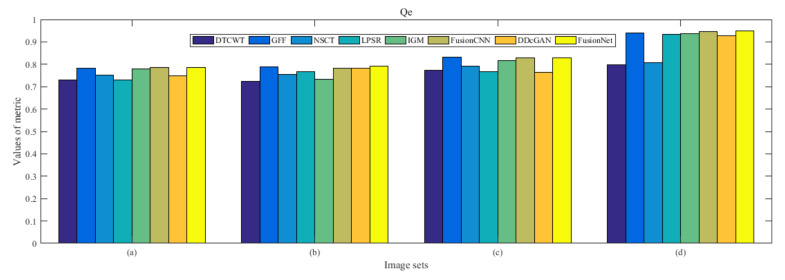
Values of $Q_{e}$ in the fused images (MRI-FDG): (a) is composed of eight images in Figure 25, (b) is composed of eight images in Figure 26, (c) is composed of eight images in Figure 27, (d) is composed of eight images in Figure 28.
